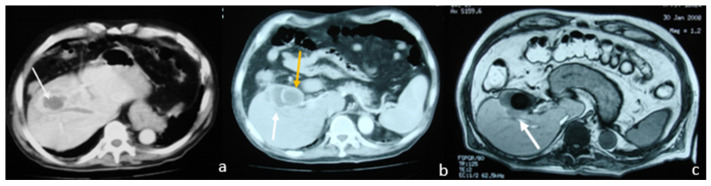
Contrast-enhanced Computed Tomography (CT) and MRI images which show overdistended and inflammatory gallbladder and a nodular lesion occupying the liver. (a) Contrast-enhanced CT: nonhomogeneous, hypodense mass located in the right lobe of the liver (white arrow); (b) contrast-enhanced CT: gallbladder with wall thickening and a large stone (yellow arrow) causing gallbladder neck obstruction (white arrow: liver abscess); (c) T1 out-of-phase axial MRI image: nodular lesion (white arrow) occupying the fourth and eighth liver segments in continuation with the overdistended and inflammatory gallbladder. It also revealed diffuse mural thickening with alternating layers of abnormal signal, as well as pericholecystic and perihepatic fluid.
